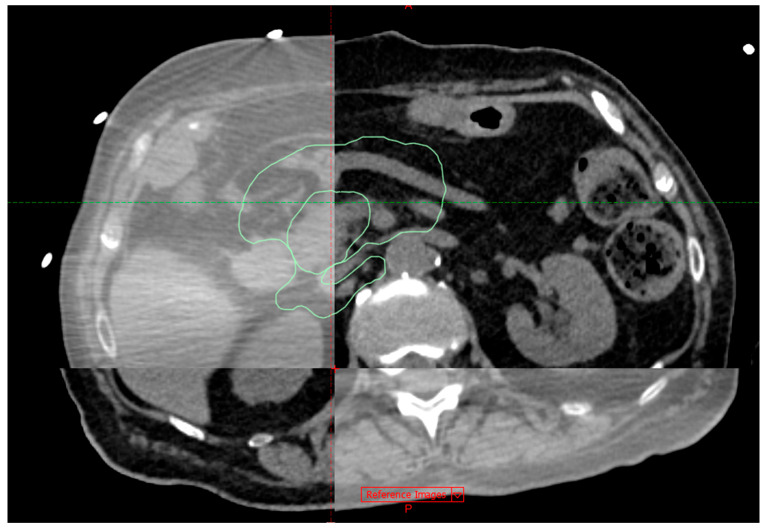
Planning MSCT co-registration with CBCT on a treatment table with the PTV conture (green) displayed.
